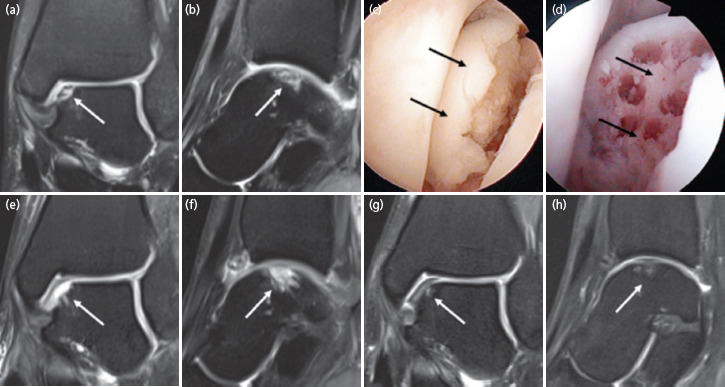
(a) Coronal and (b) sagittal MRI of the left ankle showing osteochondral lesion at the medial aspect of the talar dome (white arrows) from case 1. (c) Arthroscopic view over the medial aspect of the left ankle joint, showing the loose osteochondral fragment (black arrows). (d) View after removal of the osteochondral fragment followed by subchondral drilling (black arrows). MRI of the left ankle performed one day after surgery, with (e) coronal and (f) sagittal views following removal of the loose osteochondral fragment and subchondral drilling (white arrows) without bone grafting. Corresponding images at 2 years, (g) and (h) showing repair and regeneration of the bone and cartilage components (white arrows). These are fat-suppressed proton density-weighted MR images.
